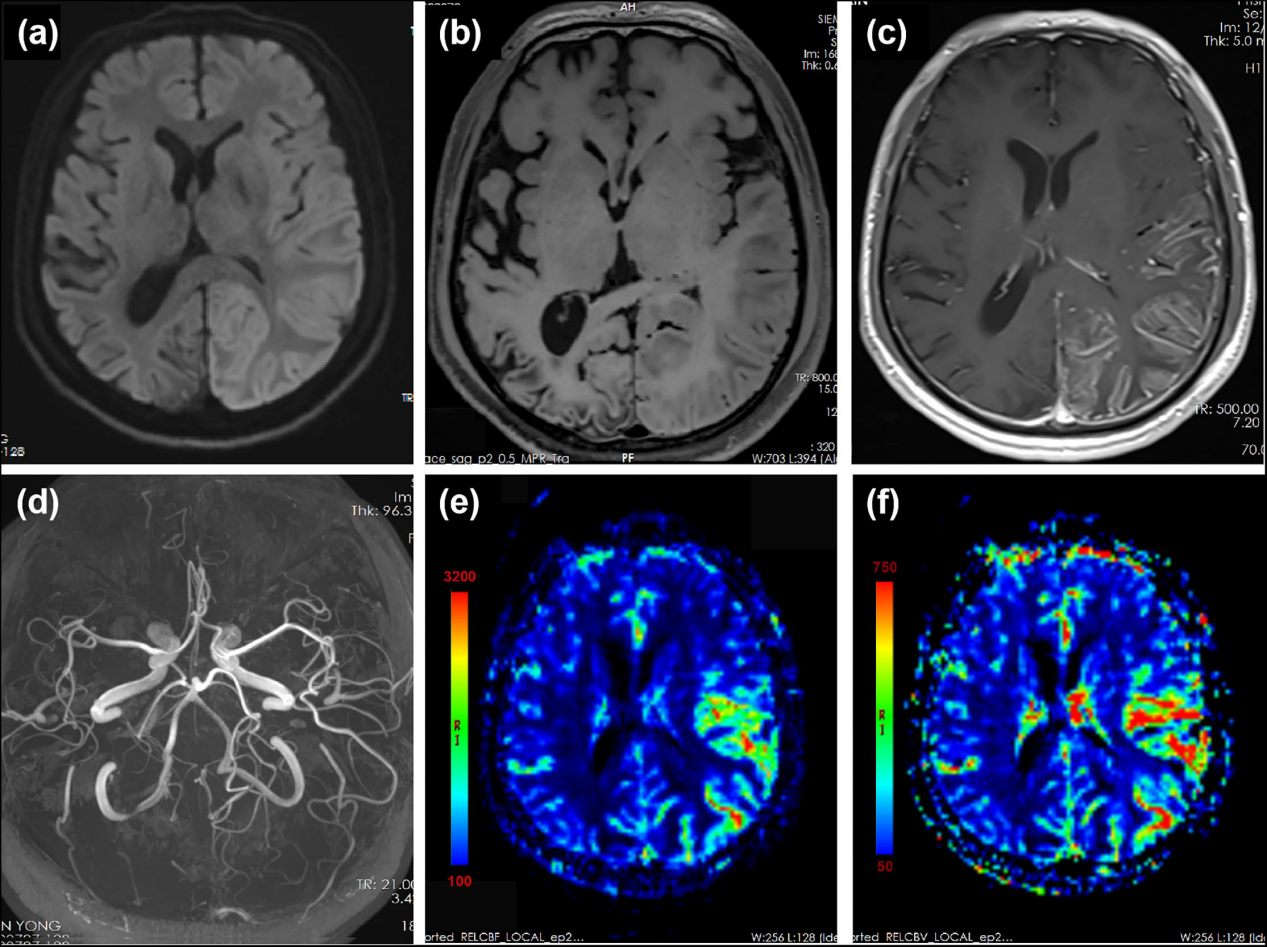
MRI performed 15 days after the onset of the second prolonged attack. DWI showed extensive cortical edema with diffusion restriction involving the left hemisphere (a). T1-weighted images showed slight hyperintensity in left temporal–occipital lobe and volume loss of right temporal–occipital lobe, suggestive of subacute and chronic stage of cortical necrosis, respectively (b). Gadolinium-enhanced T1-weighted images showed cortical enhancement in the left temporal–occipital lobe (c). MRA showed dilation of left MCA and PCA (d). PWI showed increased cerebral blood flow (CBF) (e) and cerebral blood volume (CBV) (f) in the left hemisphere. Region of interest (ROI) placed on the left temporal–parietal area showed a mean relative CBF value of 486.1 compared with that of 123.1 on the right temporal–parietal area. ROI placed on the left temporal–parietal area showed a mean relative CBV value of 533.1 compared with that of 129.8 on the right temporal–parietal area.
